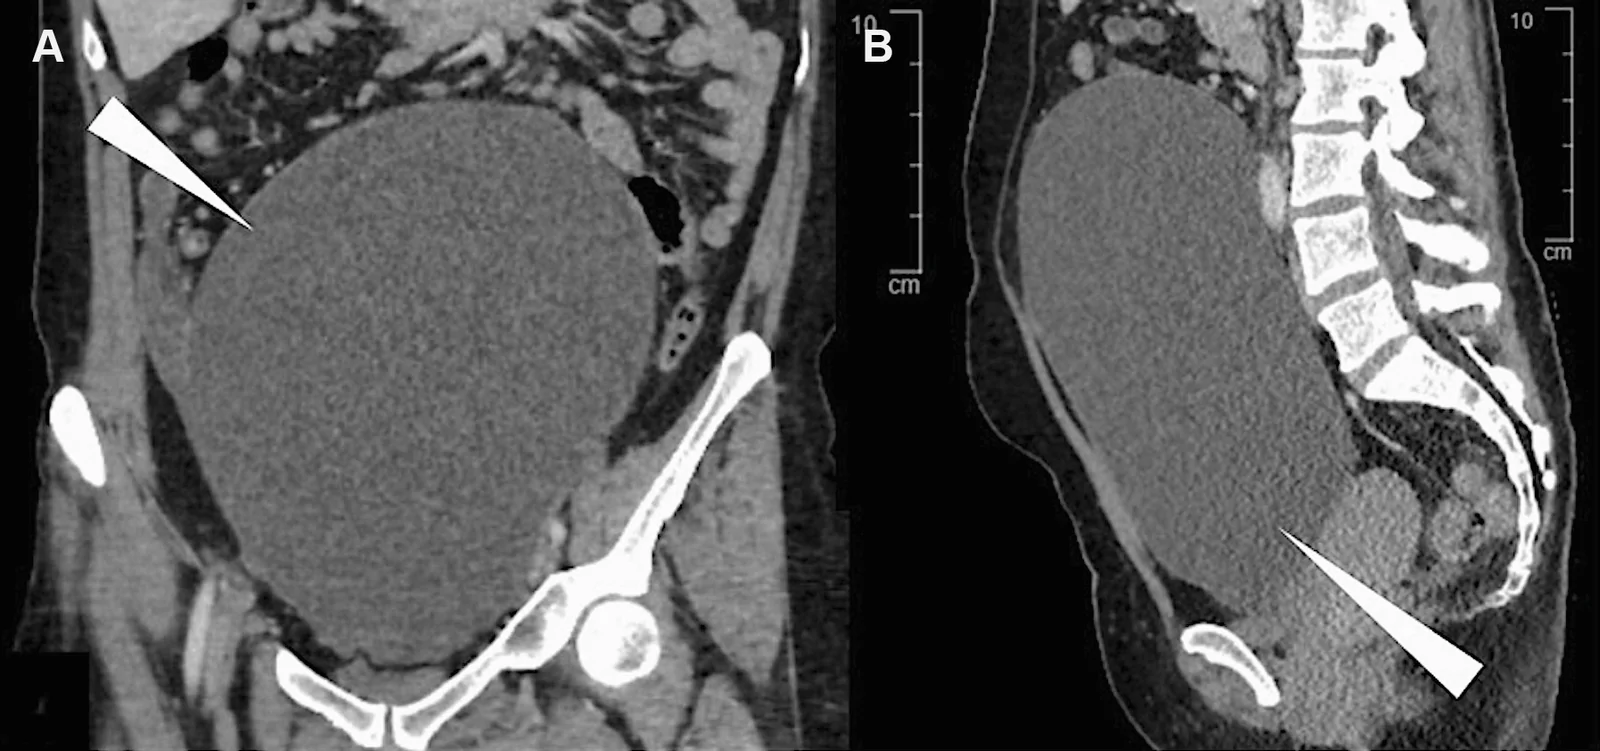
CT abdomen and pelvis with contrast in (A) coronal and (B) sagittal views, showing distended suprapubic mass (white arrowheads). CT, computed tomography.
